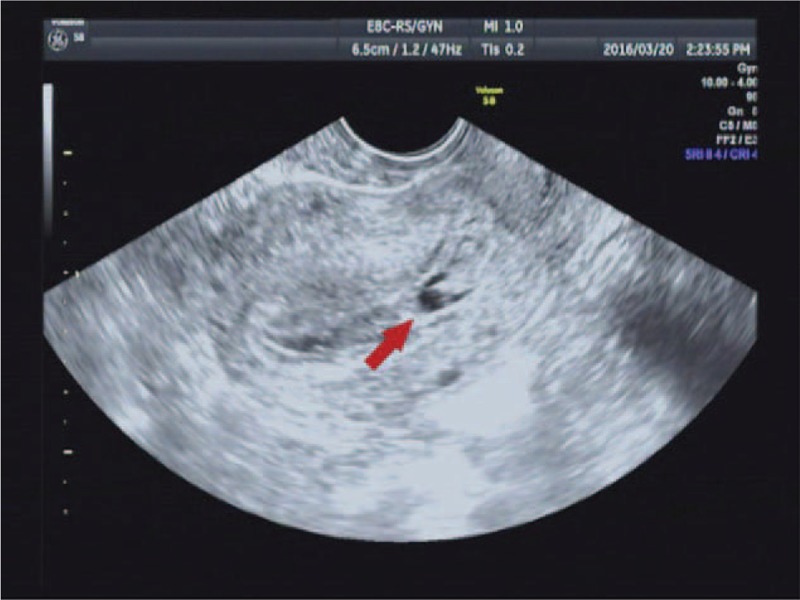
Transvaginal sonogram showing a gestational sac implanted near the previous cesarean scar in the anterior wall of the uterine corpus.
